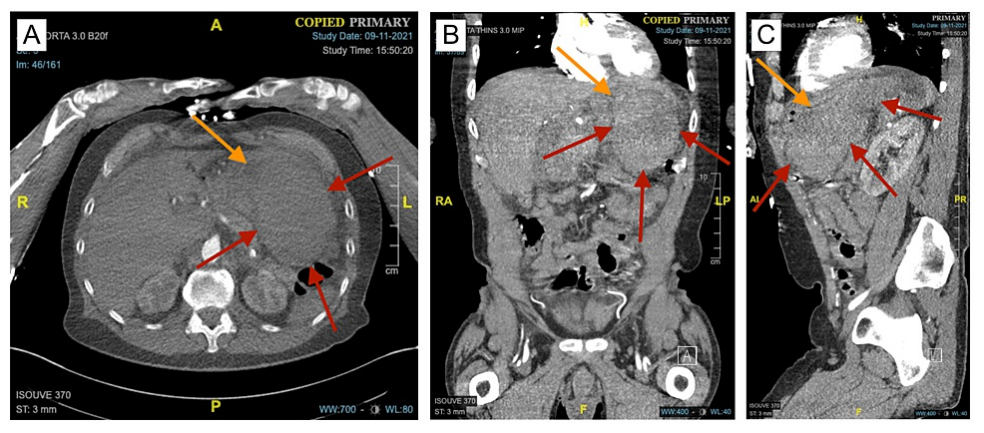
CT angiogram revealing a GIH A, B, C: Axial, coronal, and sagittal views of the initial presentation of the GIH (red arrows). The GIH was imparting a mass effect on the stomach and surrounding structures, compressing the gastric lumen (orange arrows). GIH: gastric intramural hematoma
